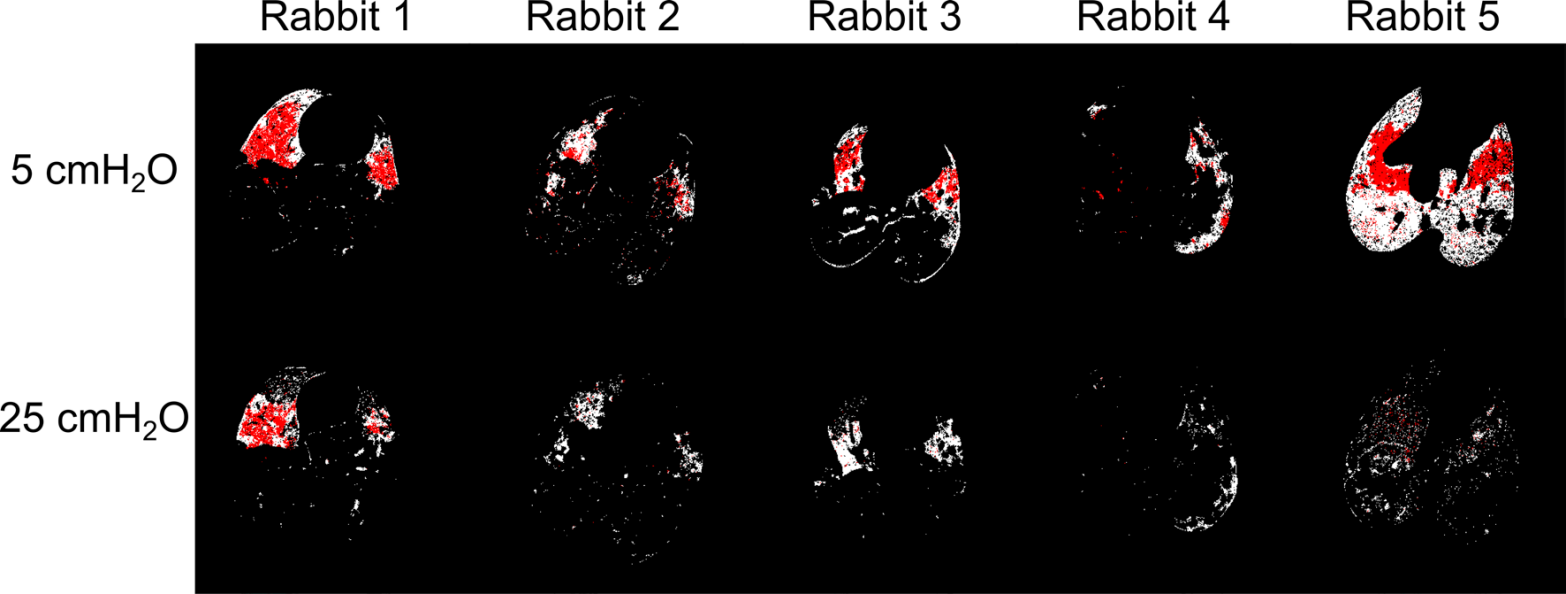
Maps of non-aerated lung regions after injurious ventilation. The maps refer to the static CT images acquired during expiration (5 cmH_2_O, upper panel) and inspiration (25 cmH_2_O, lower panel). White: non aerated regions after injurious ventilation; Red: non aerated regions after injurious ventilation which were already present after saline lavage. A spatial co-localization between non-aerated regions before and after injurious ventilation is visible at a pressure of 5 cmH_2_O. Recruitment of non-aerated regions upon inspiration is visible after saline lavage (lower panel).
